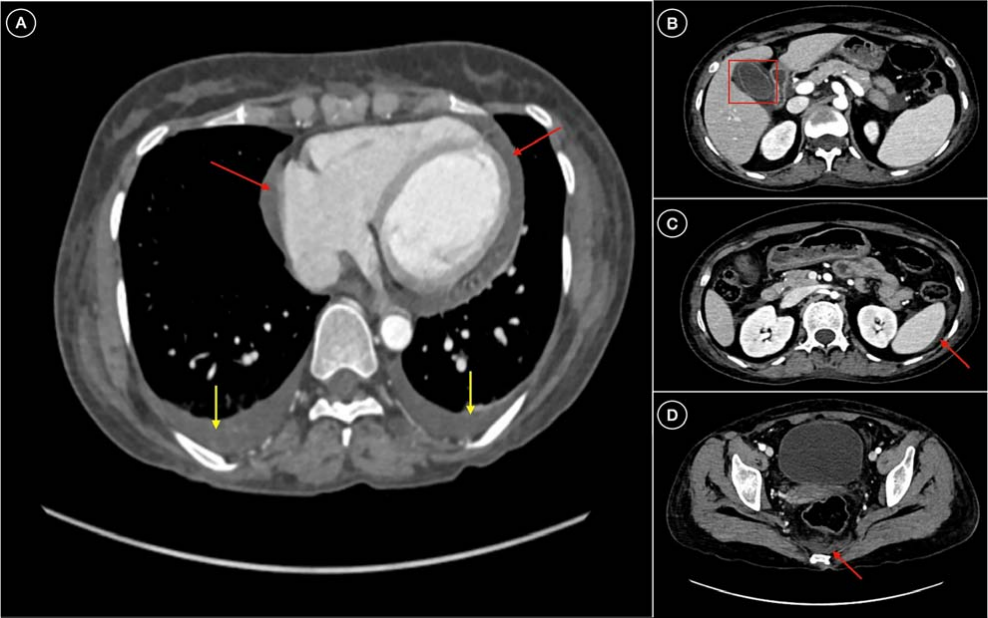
Axial contrast-enhanced computed tomography of the abdomen shows (A) pericardial effusion (red arrows) and bilateral pleural effusion (yellow arrows); (B) edematous gallbladder wall with surrounding pericholecystic fluid (acalculous cholecystitis) (red box); (C, D) minimal fluid in the pelvic region, perisplenic region, bilateral pararenal space, and adjacent bowel loops (red arrows).
